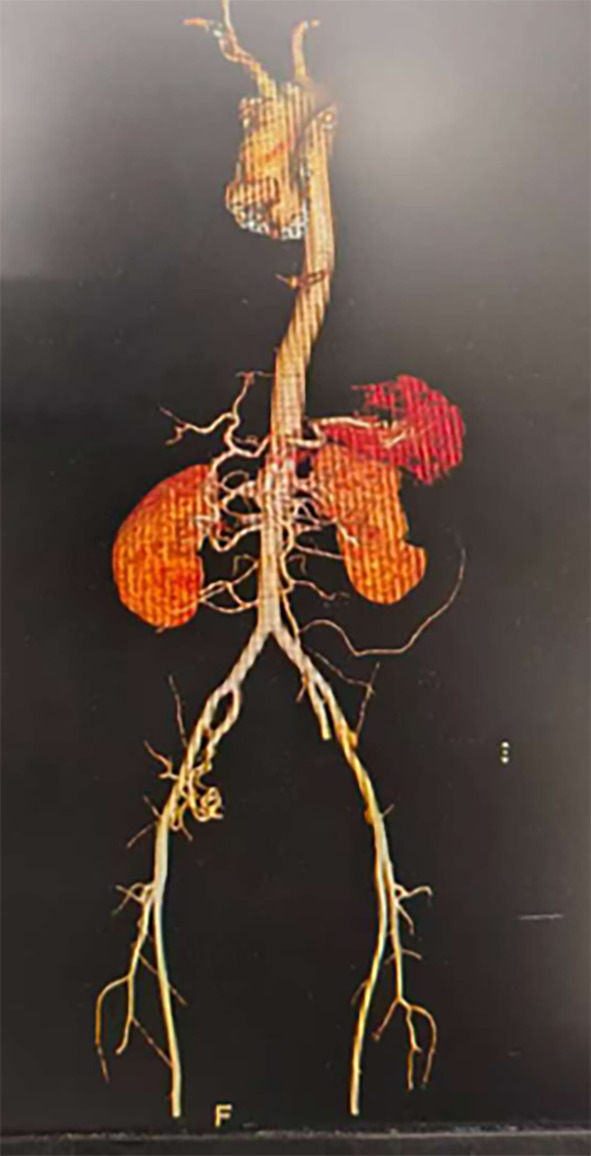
Preoperative computed tomography angiography (CTA) image of the patient.
